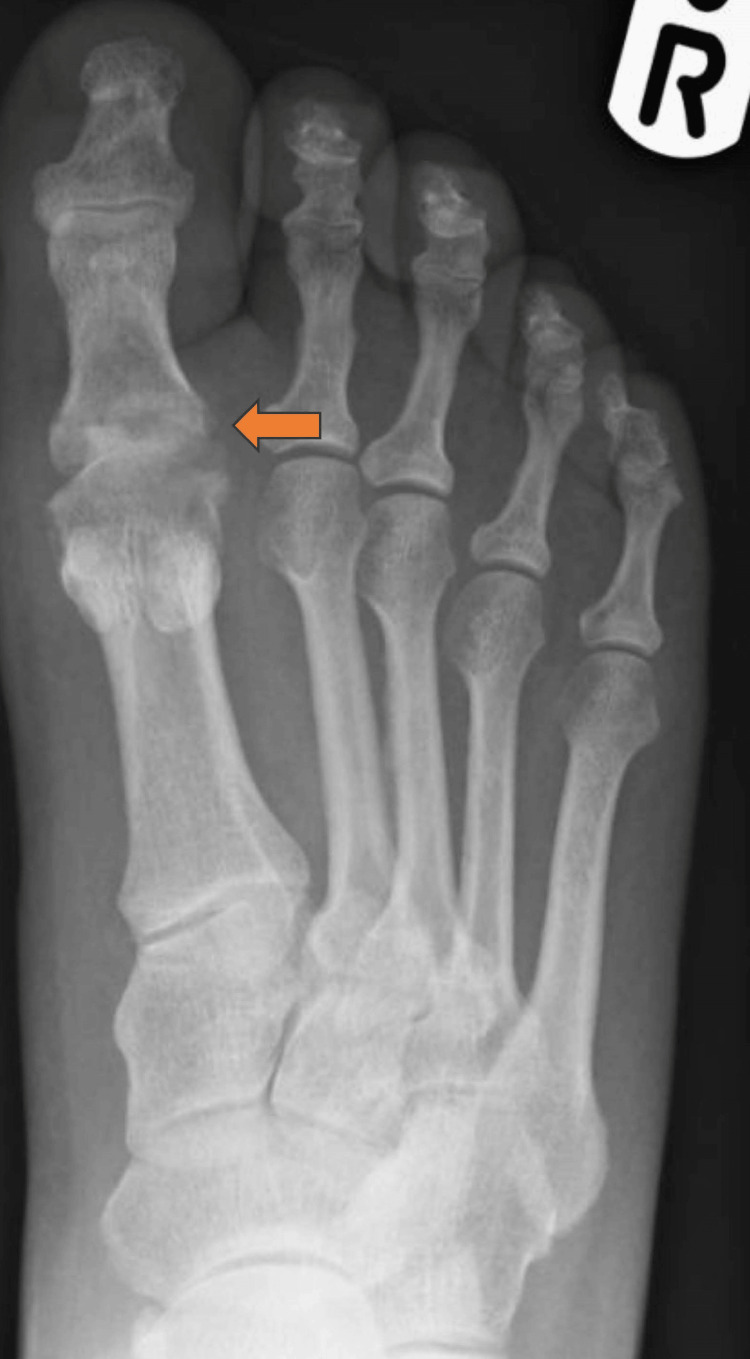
Radiograph Showing Infected First Metatarsophalangeal Joint Replacement
